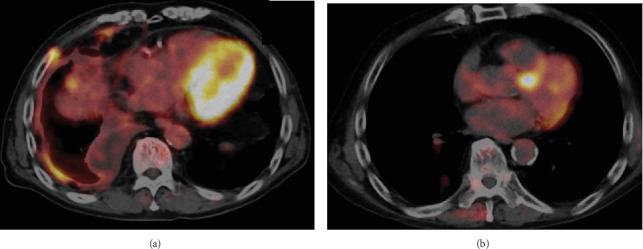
PET-CT scan (a) before ICI therapy and (b) after 1 year. Complete response (CR) was achieved. ICI, immune checkpoint inhibitor.
